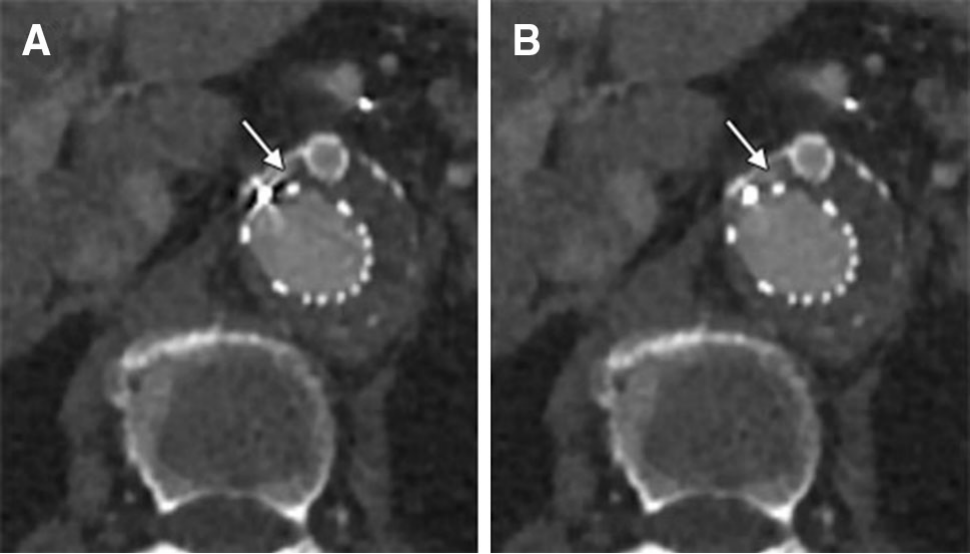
CT images (A standard (AIDR 3DE, B SEMAR) of a type 1 endoleak in a 70-year-old female. A small amount of contrast is seen immediately ventral of the right renal artery fenestration. The endoleak is visible on both the standard and SEMAR image, but is better appreciated on the SEMAR image (arrow)
